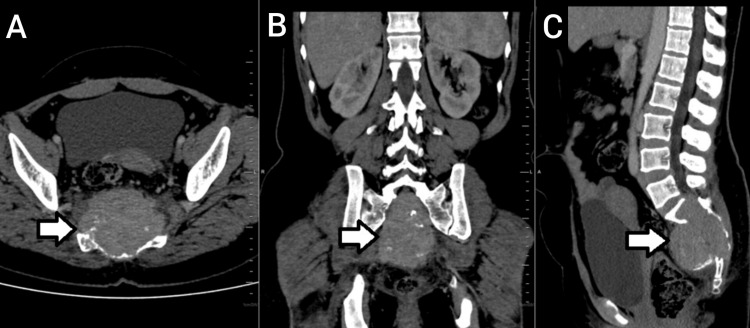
Lumbopelvic CT scan demonstrating a large, well-defined expansile lytic lesion (arrow) involving the sacrum (S1-S5) with extension into the presacral and sacral spaces, presented in (A) axial, (B) coronal, and (C) sagittal views
